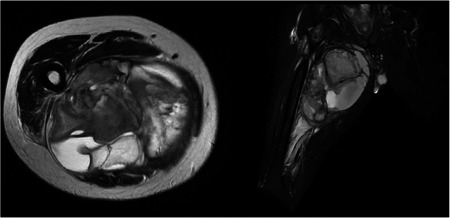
Magnetic resonance images of a wide synovial sarcoma localized in the thigh.
